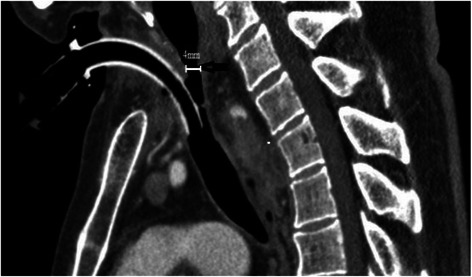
Tracheal stenosis in Case 2: A computed tomographic scan image of a 62-year-old man (*case 2*) showing severe tracheal stenosis with diameter of 4 mm above the site of tracheostomy (*arrow*)
